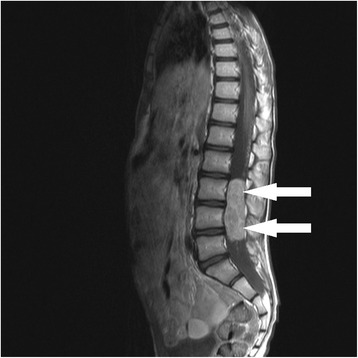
MRI of the spine. Sagittal contrast enhanced T1- weighted image shows a well-circumscribed, ovoid, strong enhancing intradural extramedullary mass at the L3-L4/5 level
